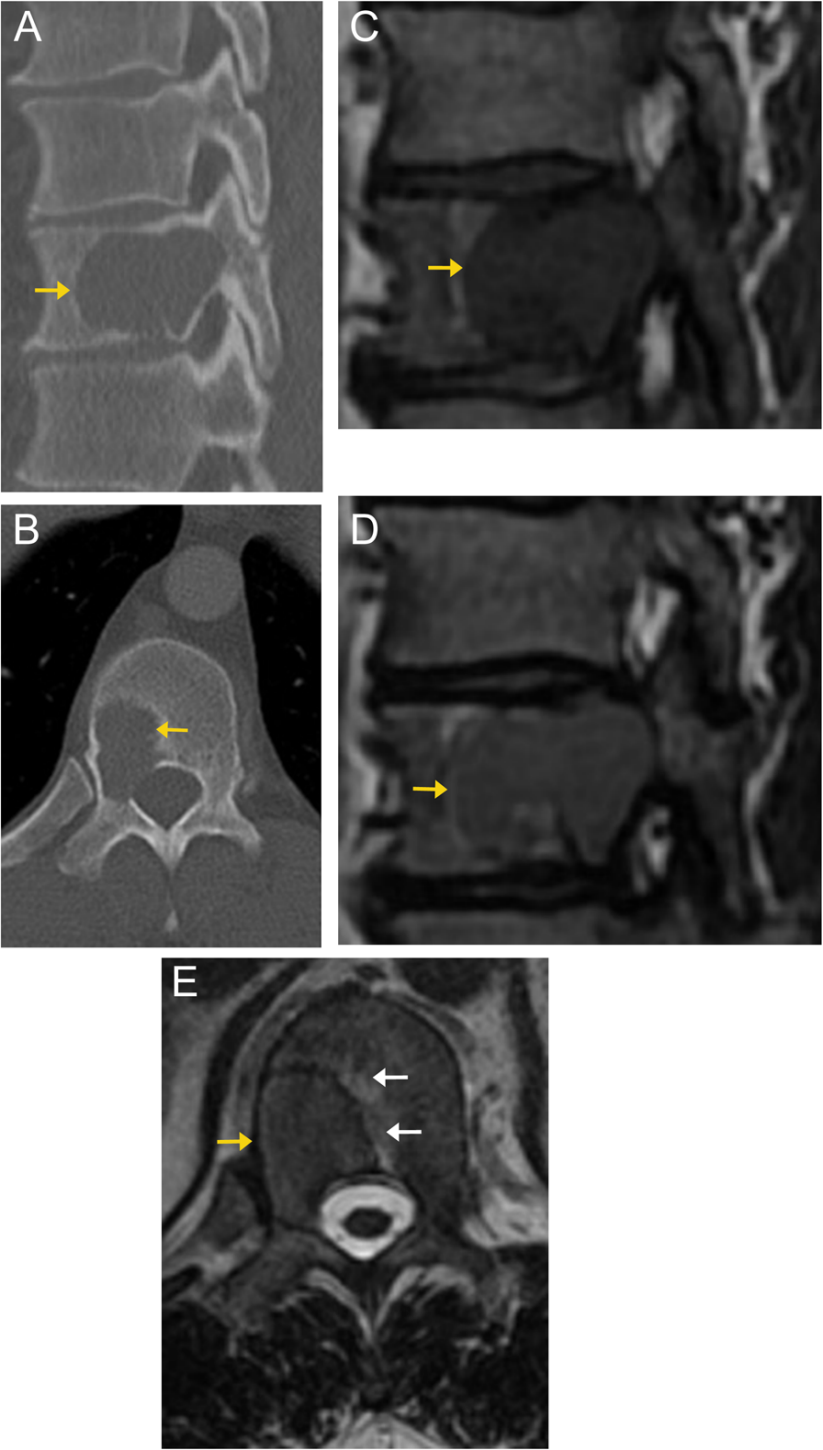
Giant cell tumor. Sagittal (a) and axial (b) CT images demonstrate a well-defined, expansile lytic lesion involving the right posterolateral aspect of the T10 vertebral body and right pedicle. No discrete sclerotic rim is seen around the lesion margins.  Sagittal and axial MR images demonstrate a homogenous, well defined mass (orange arrows) in the posterior aspect of T10, with low signal on T1- (c, d) and low to intermediate signal characteristics on T2- (e) weighted sequences. A trace amount of increased signal is seen in the adjacent marrow, consistent with mild marrow edema (white arrows)
